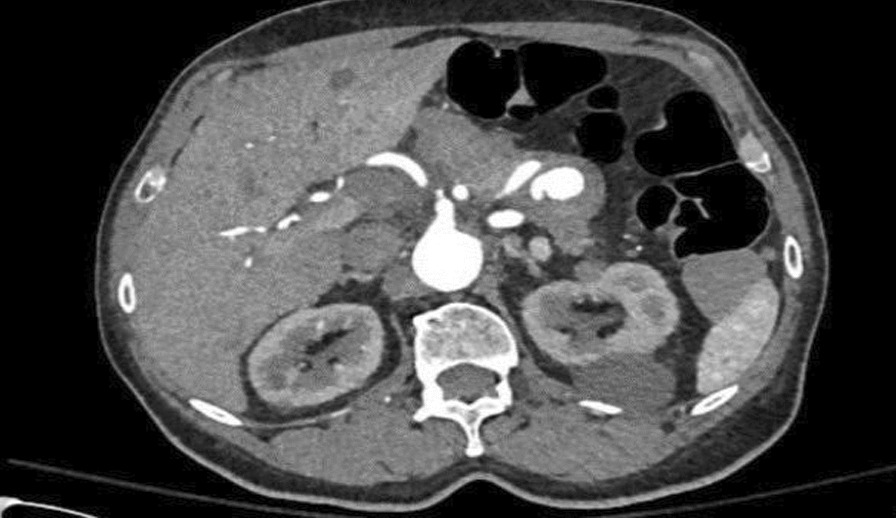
Computed tomography of the abdomen showing splenic artery pseudoaneurysm
